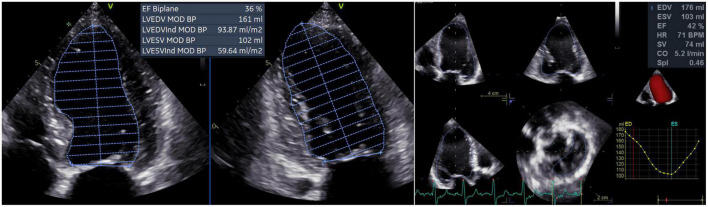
Left ventricular volumes and ejection fraction calculated by two-dimensional echocardiography (left panel) and measured by three-dimensional echocardiography (right panel). CO, cardiac output; EDV, left ventricular end-diastolic volume; ESV, left ventricular end-systolic volume; EF, ejection fraction; HR, heart rate; LV, left ventricular; MOD BP, biplane mode; SV, stroke volume; SpI, sphericity index.
